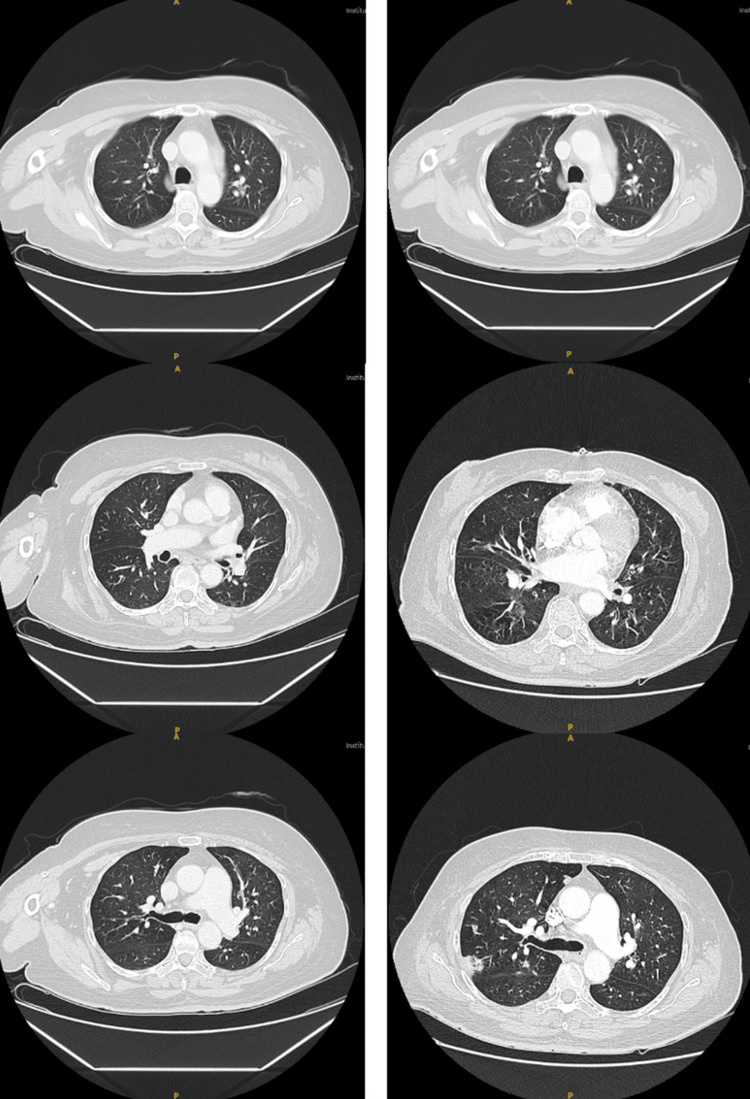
CT images of the chest. The scan obtained in June 2023 (left) shows no evidence of pulmonary lesions, while the follow-up scan (right) demonstrates metastatic nodules in the lungs.
